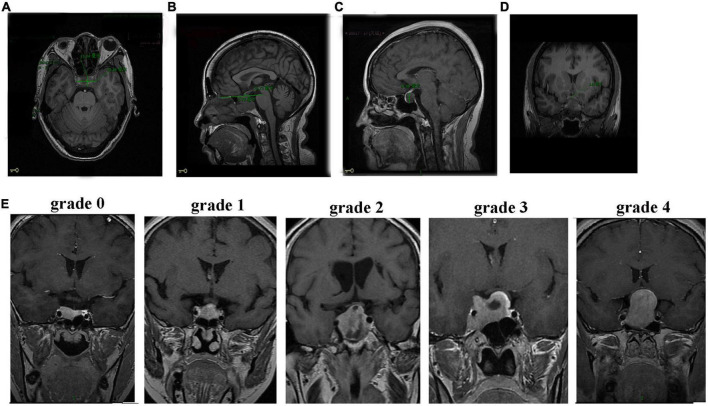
MRI images of patients with NFPA. (A) Axial position measurement of the horizontal diameter line (30.58 mm) and the anteroposterior diameter line (15.04 mm). (B) Sagittal measurements of the epitaxial height of the sella turcica diameter line (3.79 mm). (C) Sagittal measurements of the vertical diameter line (14.99 mm). (D) Thickness measurement of the optic chiasm. (E) Grades (0–4) of tumor compression on the optic chiasm.
